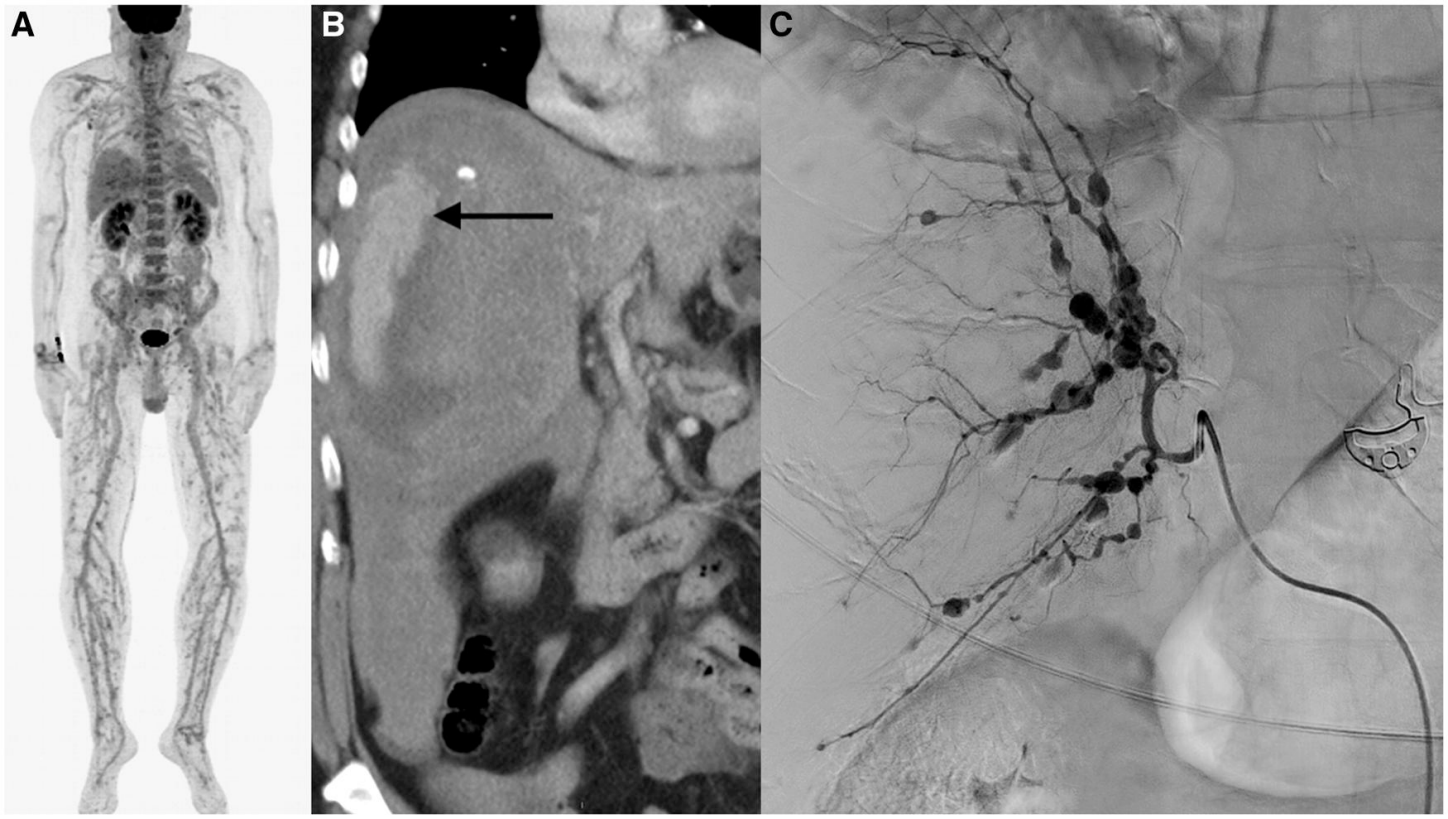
(A) PET/CT. The maximum-intensity projection shows increased FDG uptake in the peri- and intramuscular arterial tree of the lower extremities and inhomogeneous uptake in the liver. (B) CT of the abdomen, coronal view. Haematoma in the right lobe of the liver (arrow). (C) Angiography of the hepatic artery showing microaneurysms and stenoses
